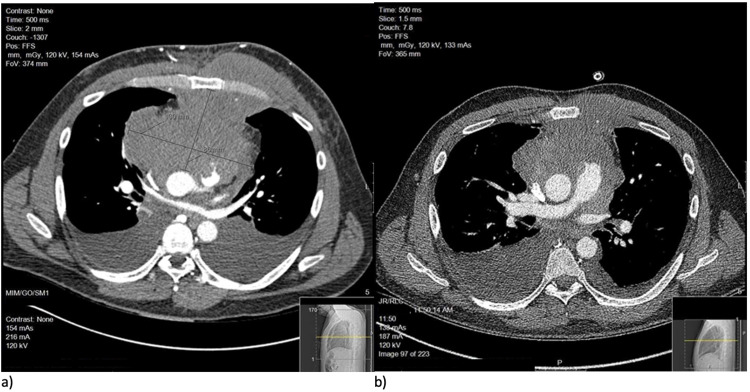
Comparative image set showcasing a pre-treatment CT thorax axial cut showing extensive mediastinal mass (a) versus CT-thorax axial cut at approximately the same level after five cycles of R-CEOP (b). CT: computed tomography.
